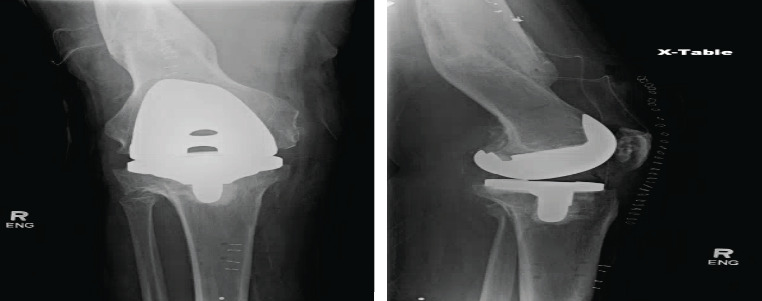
Postoperative anteroposterior and lateral X-ray views of the status of postrobotic arm-assisted total knee arthroplasty and removal of high tibial osteotomy screws.
